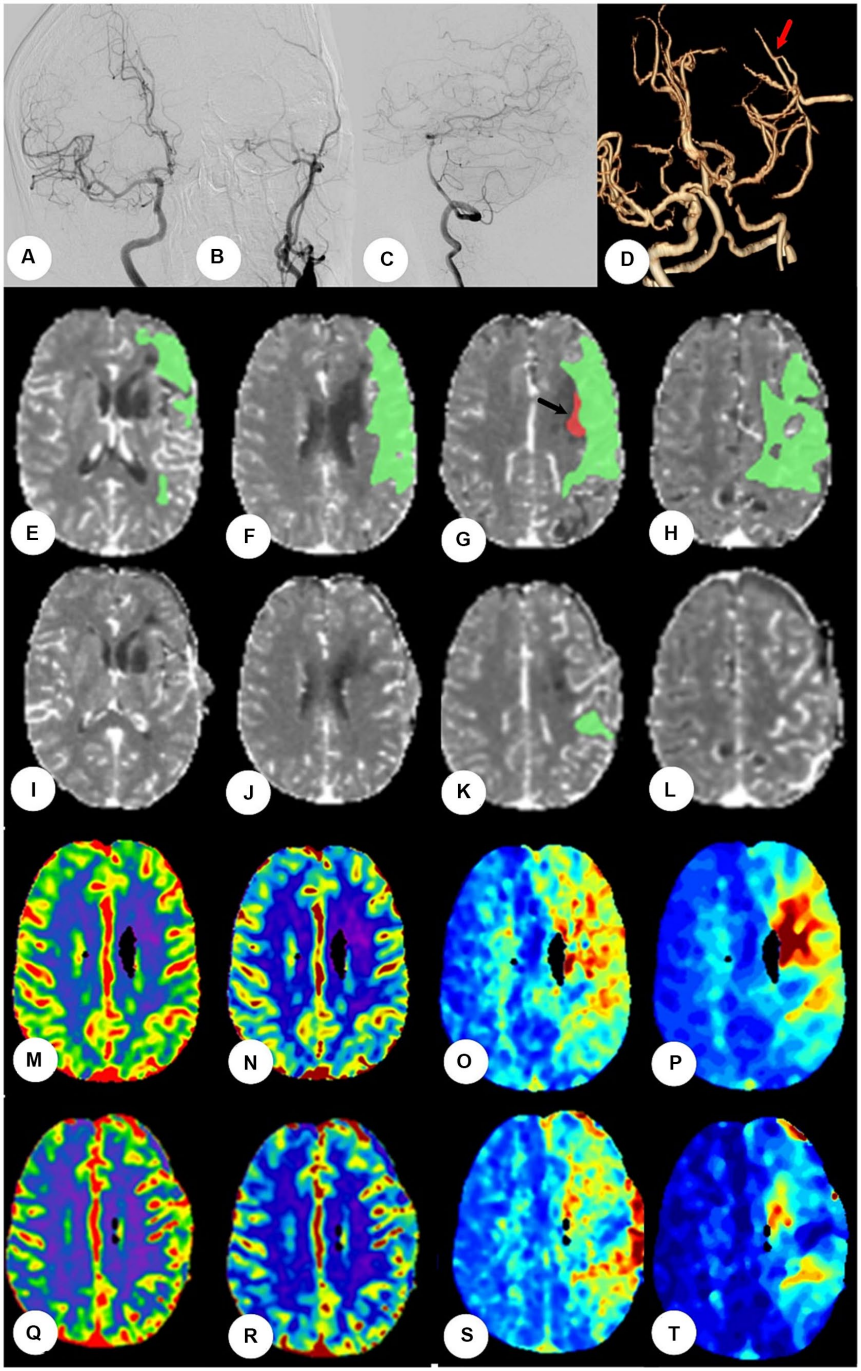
The patients were performed by CTP at the fourth day after bypass surgery. (A–C) Digital subtraction angiography showed left initial internal carotid artery was occluded, right the left internal carotid artery and bilateral vertebral artery systems is normal, but without obvious compensation. (D) Postoperative CT angiography showed the blood flow at anastomosis was patent (red arrow). (E–H): Preoperative CTP showed the mismatch green area (Tmax>6 s) was 93.1 mL and the hypoperfusion red area (rCBF<30%) was 0.6 mL at frontotemporal parietal lobe (black arrow). (I–L) Postoperative CTP showed mismatch area reduced significantly and hypoperfusion area disappeared. (M–P) Figures of CTP showed CBV, CBF, and MTT and Tmax, respectively, before bypass. The values of CBV and CBF were normal, the values of MTT and Tmax lengthened at left frontotemporal parietal lobe. (Q–T) The values of CBV and CBF were still normal, and the values of MTT and Tmax shortened significantly after bypass surgery.
